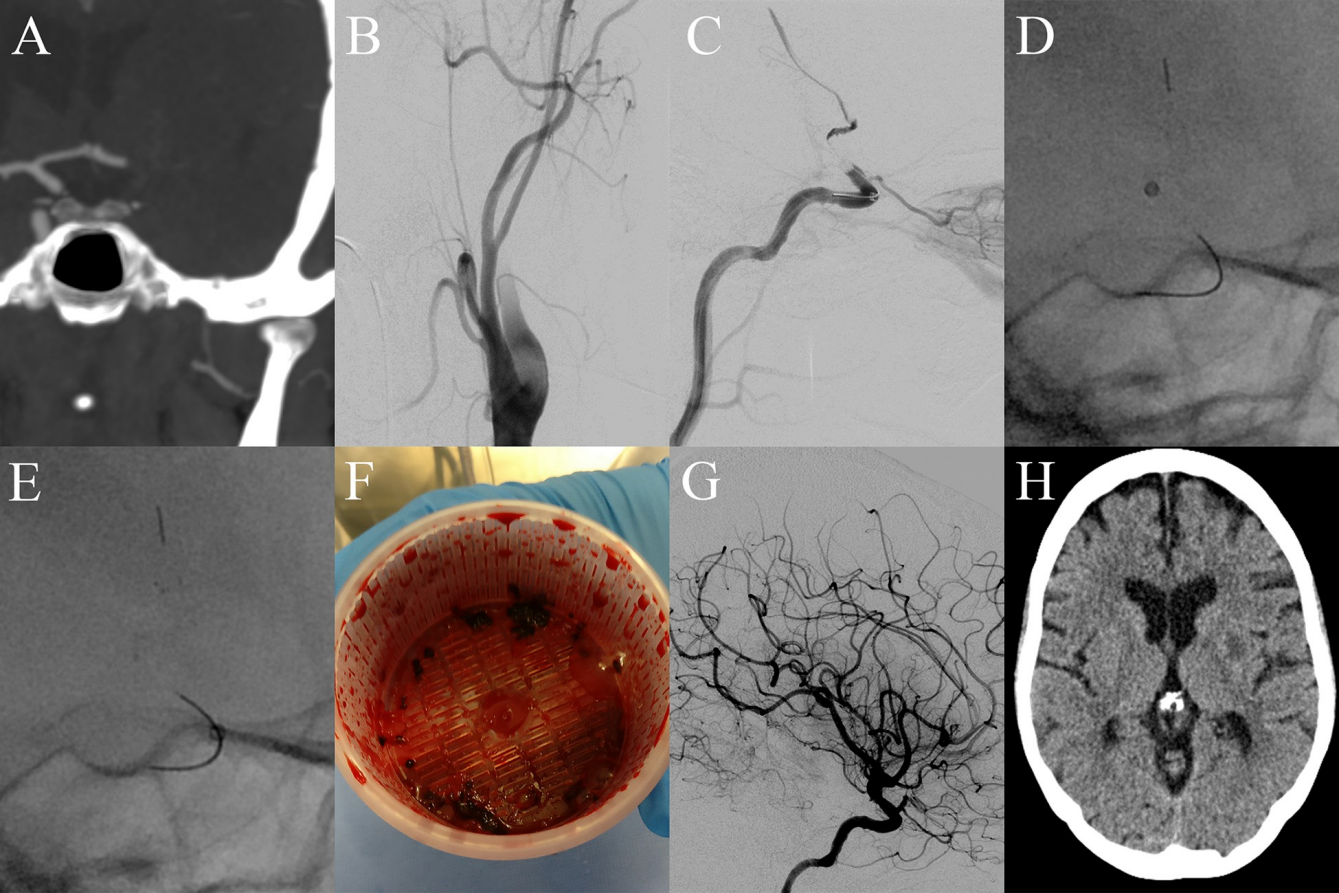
Endovascular treatment with mSAVE. A, CT-angiography (coronal view) of a patient suffering from a distal occlusion of the internal carotid artery (ICA) on the left site. B, First angiogram demonstrating extracranial pseudo-occlusion of the ICA. C, Positioning of a stent retriever in the distal ICA and proximal M1 segment with antegrade flow via the deployed stent retriever. D, The aspiration catheter is connected to an activated pump and advanced to the proximal face of the clot until vacuum is achieved. E, The aspiration catheter is then retrieved along the wire of the stent retriever without moving the stent retriever itself in order to reduce thrombus mass and subsequently advanced to the occlusion site after vacuum is lost. F, The pump canister is showing massive amount of clot prior to embolectomy. G, After performing one attempt of SAVE maneuver the intracranial lesion is recanalized and the downstream territory completely reperfused. H, Final computed tomography at discharge demonstrating a small infarction within the basal ganglia.
